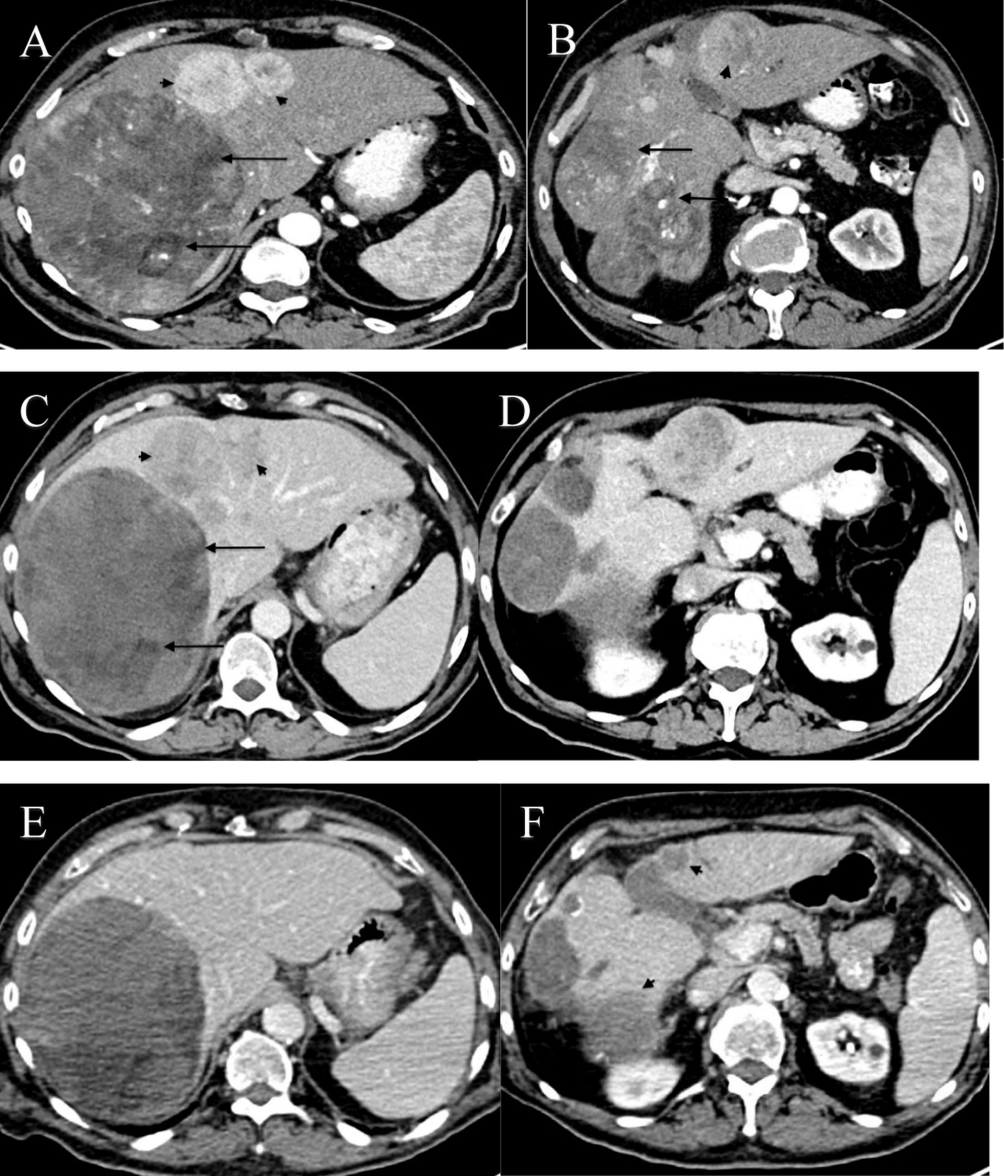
(A–F) Axial CT images of the abdomen at two levels through the liver showing multiple intraparenchymal masses with imaging features consistent with HCC, obtained during different stages of treatment. (A,B) Axial CT images at two levels before initiation of treatment, during the early arterial phase. (A) CT scan demonstrates a large anterior enhancing lesion in the right lobe of the liver with a mosaic pattern and low-density areas consistent with necrosis and fatty material (black arrows). Additional smaller arterial enhancing lesions were noted in the left lobe (black arrowheads). The liver did not demonstrate a cirrhotic pattern, prompting a biopsy. No portal venous involvement was noted. (B) A lower image through the liver shows lobulated heterogeneous masses (black arrow) in segments VI/VII and a well-defined mass with strong enhancement (arrowhead). (C,D) Axial CT images at the same levels, three months after initiation of treatment with atezolizumab and bevacizumab, obtained during the late arterial phase. Images demonstrate low attenuation areas in the peripheral portion of segment IV (black arrows) and two heterogeneous enhancement masses with no significant difference in size (black arrowheads). (E,F) Axial CT images at the same levels, two years after initiation of treatment with IV Vitamin C, obtained during the porto-venous phase. Images show an interval decrease in size (black arrowheads) and resolution of some lesions, suggestive of a complete response.
